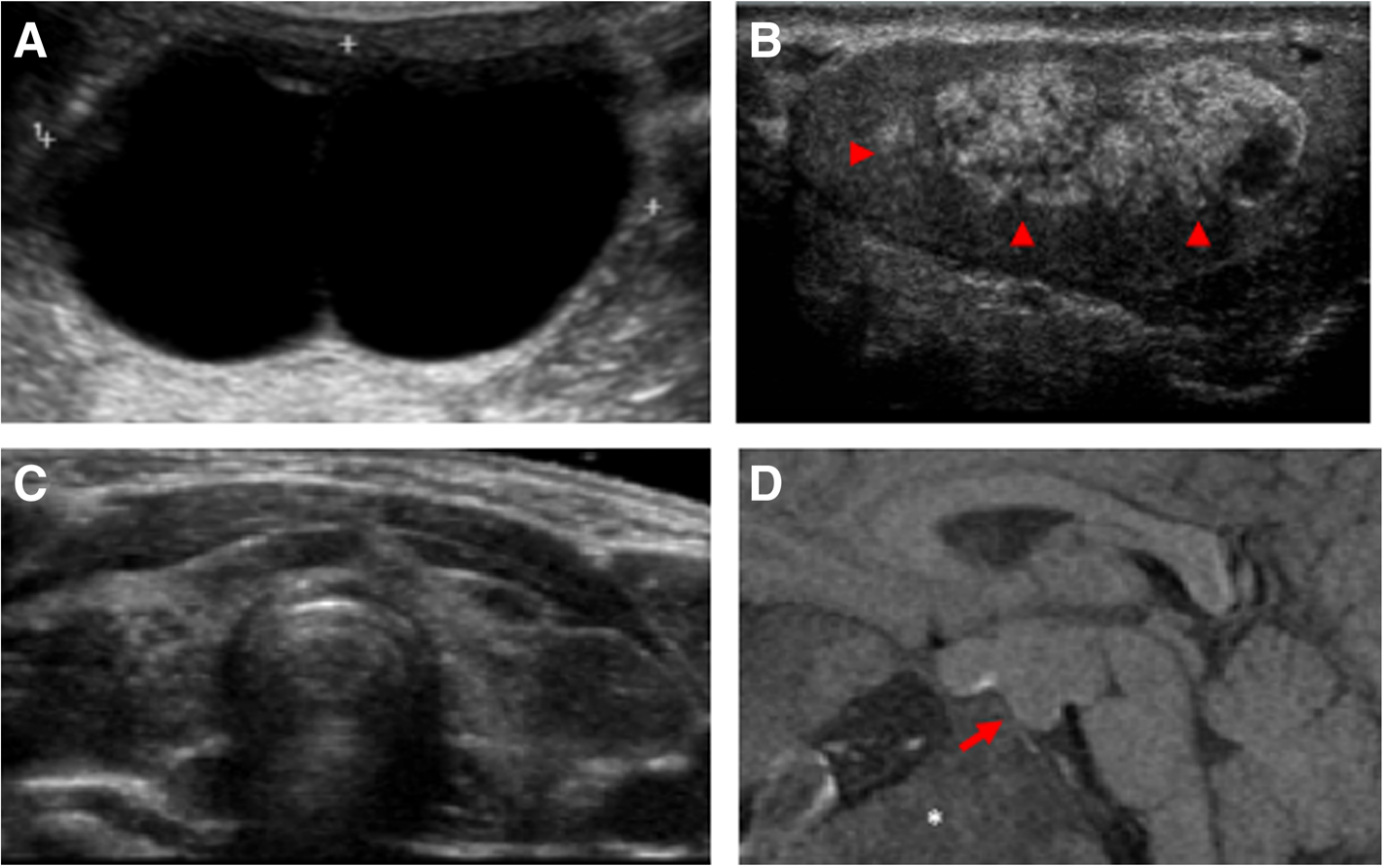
Representative radiographic features of endocrine involvement in McCune-Albright syndrome. a Pelvic ultrasonography in a 5-year-old girl with clinical signs of precocious puberty demonstrating a large unilateral ovarian cyst. b Testicular ultrasonography in a patient with macro-orchidism demonstrating a discrete, mixed hyper- and hypoechoic lesion (red arrowheads). c Thyroid ultrasonography showing diffuse, bilateral involvement with multiple hyper- and hypoechoic nodules. d A pituitary MRI in a patient with growth hormone excess revealing a pituitary macroadenoma (red arrow) and fibrous dysplasia involvement throughout the skull base (white star)
